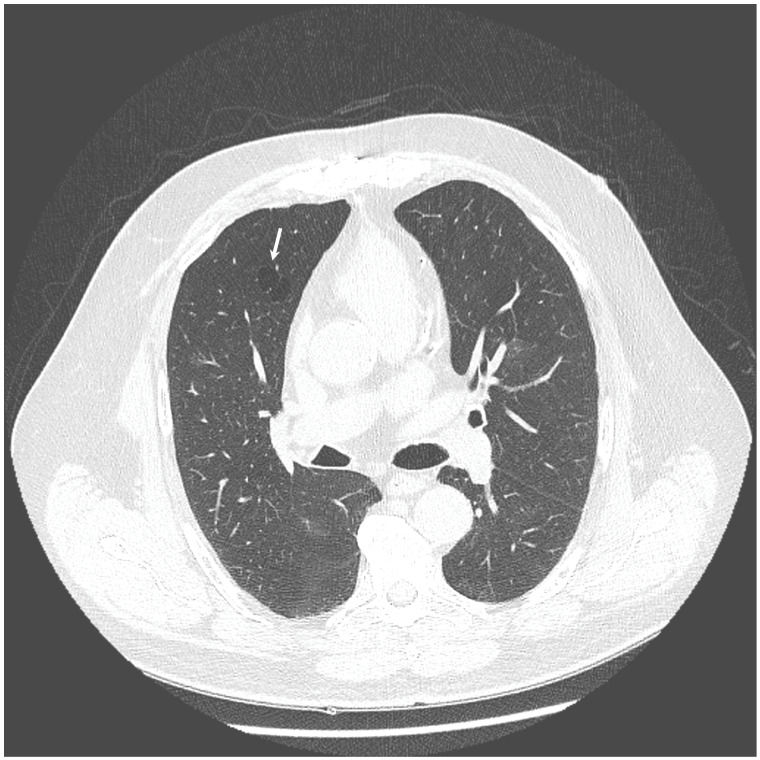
HRCT scan—Emphysema—the white arrow indicates the emphysematous changes in the lung.
